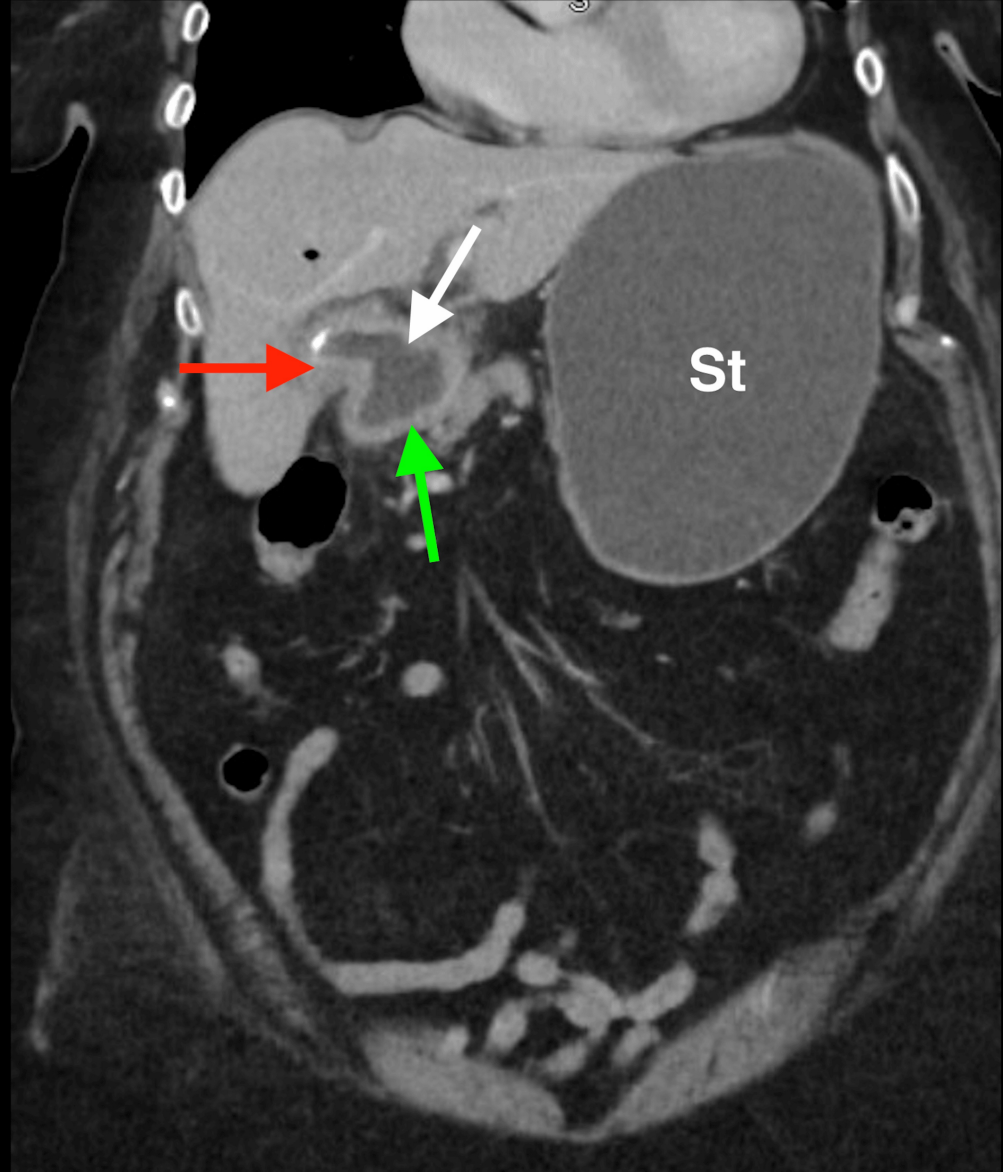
Coronal contrast-enhanced CT of the abdomen demonstrating a biliary-enteric fistula. The red arrow highlights the gallbladder, the green arrow indicates the adjacent duodenum, and the white arrow demonstrates the fistulous communication between the gallbladder and duodenal lumen, consistent with a cholecystoduodenal fistula. The stomach (St) is labeled for anatomical orientation.
